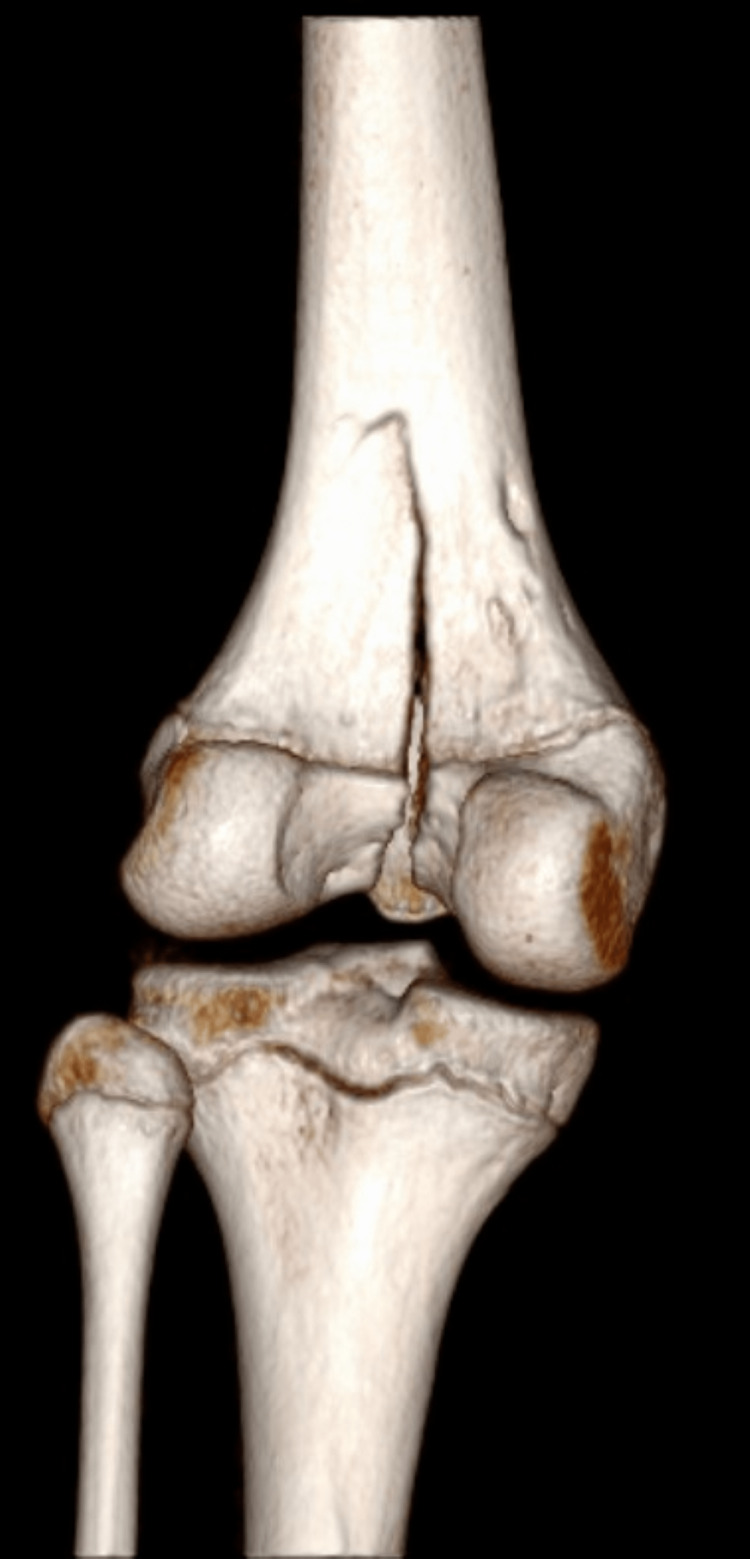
CT with 3D reconstruction of the left knee, posterior view
